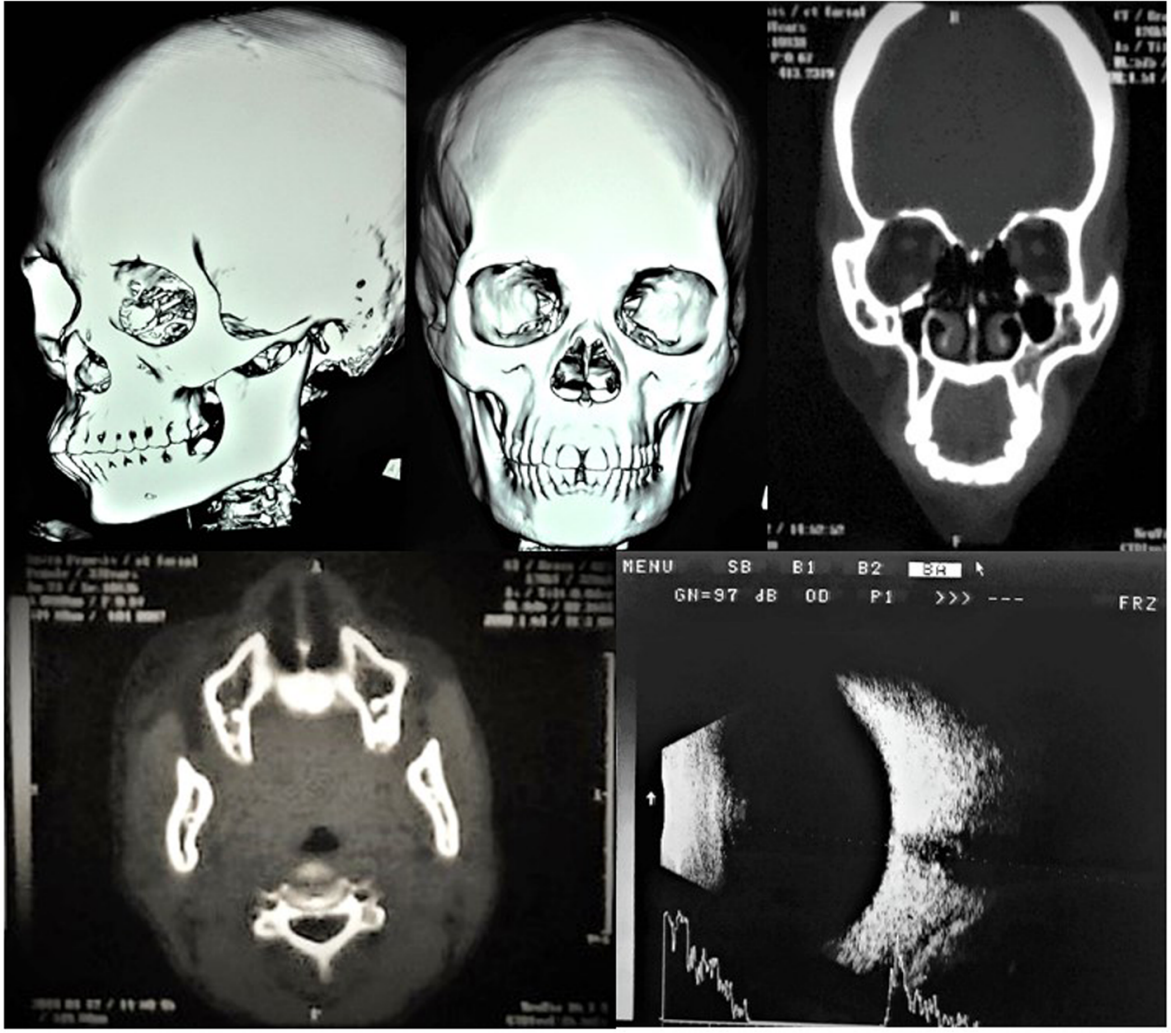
Brightness scan (ophthalmic ultrasonography) of the left eye showing no abnormalities; three-dimensional computed tomography of facial bones showing mild left zygomatic bone involvement and computed tomography showing decreased size of orbital bones on left side together with atrophy of facial subcutaneous and masseter muscle on the affected side
